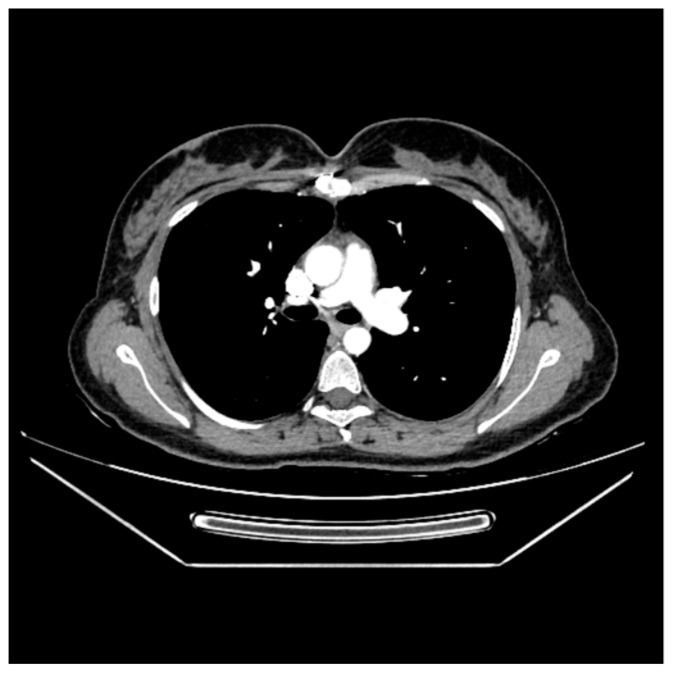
Pulmonary CT angiography at three-month follow-up, showing no pathological findings.
